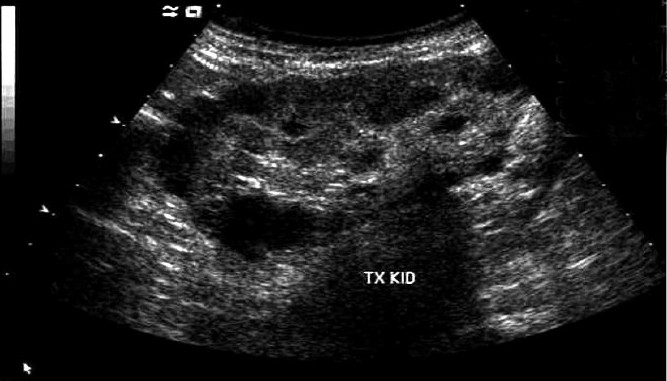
Recent ultrasonography of transplant kidney
